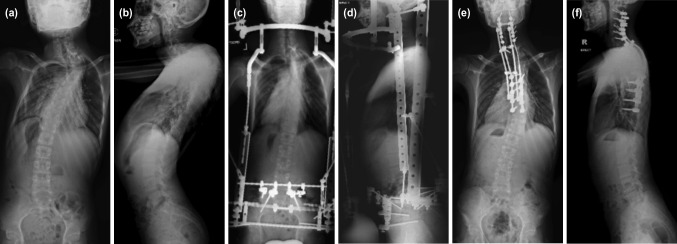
Plain radiographs (posteroanterior or and lateral view) of Case 1: (a, b) before operation, (c, d) while on halo-pelvic traction, and (e, f) after single-stage posterior spinal fusion.
